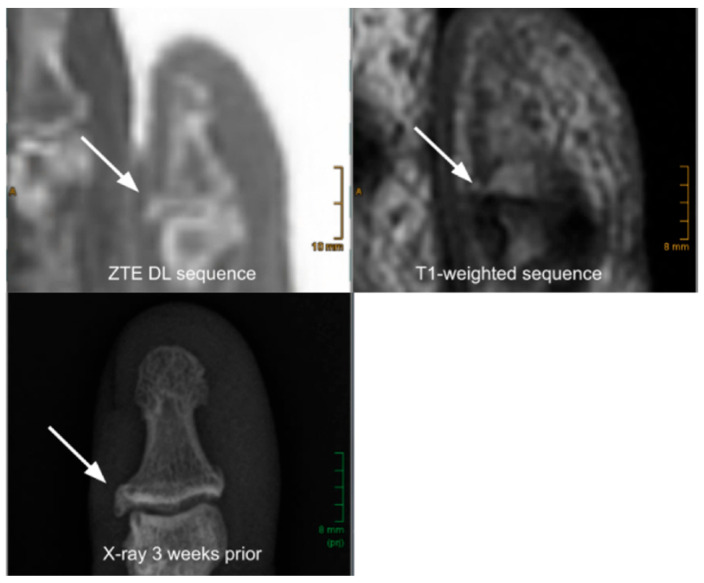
Sixty-year-old male with osteoarthritis of the hand and pain in the proximal and distal interphalangeal joints, imaged with coronal ZTE DL (left) and T1-weighted MRI (right), with a corresponding radiograph obtained 3 weeks earlier (bottom). An osteophyte (white arrow) at the distal interphalangeal joint is more clearly depicted on ZTE DL than on T1-weighted imaging.
